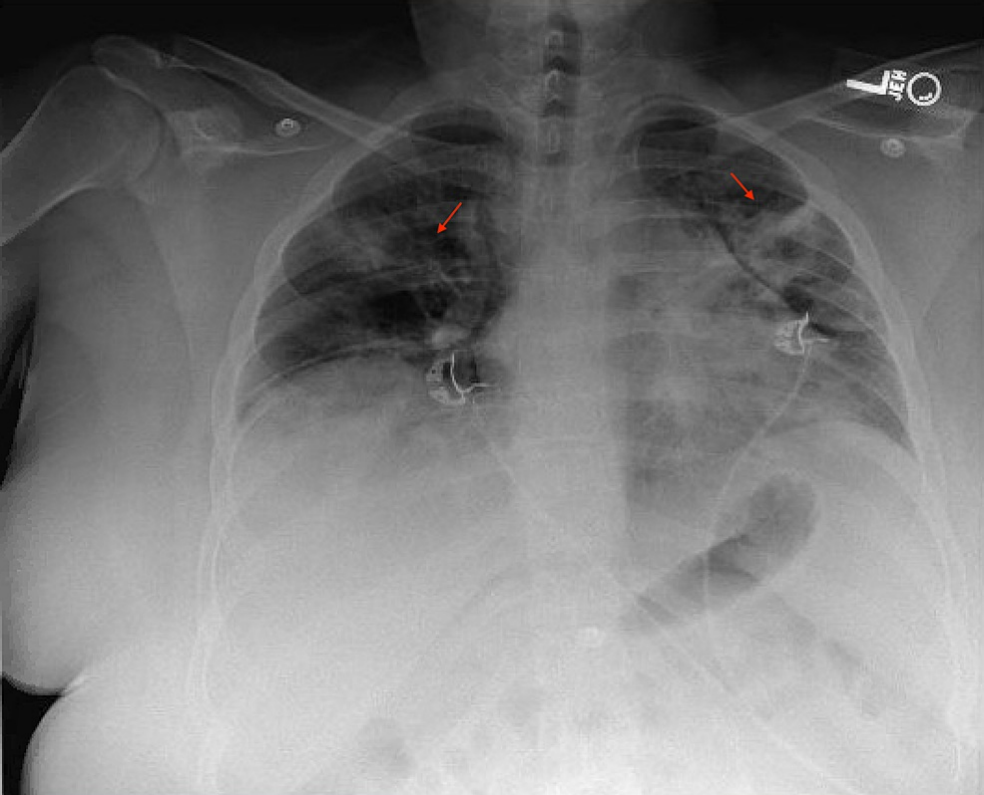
Chest radiograph showing low lung volumes with acute inflammatory infiltrates in the upper lobes accompanied by subsegmental atelectasis.
